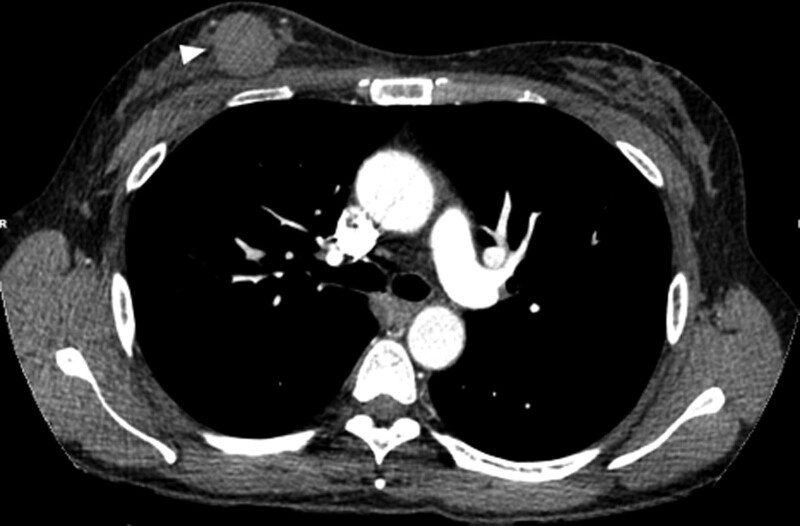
On preoperative chest CT scan, a 3.0 × 2.8 cm relatively well-defined homogeneously enhancing mass (arrowhead) is detected in the right breast. CT = computed tomography.
